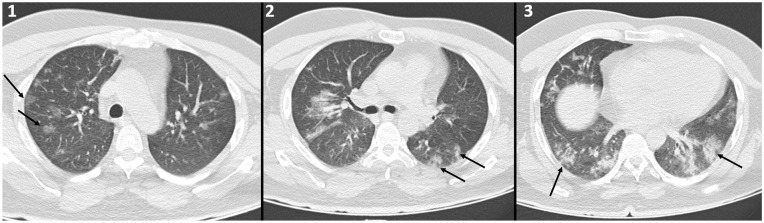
A non-contrast head CT demonstrated a cortico-subcortical hypodensity in the right
